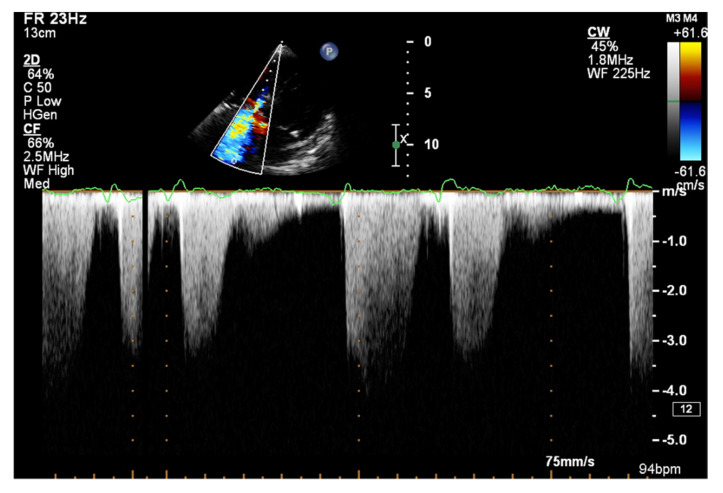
Doppler assessment of tricuspid regurgitant velocity (TRV). Differential rate of tricuspid regurgitation wave jets in atrial fibrillation. TTE, 4CH view. TTE: transthoracic echocardiography; 4CH: four-chamber.
